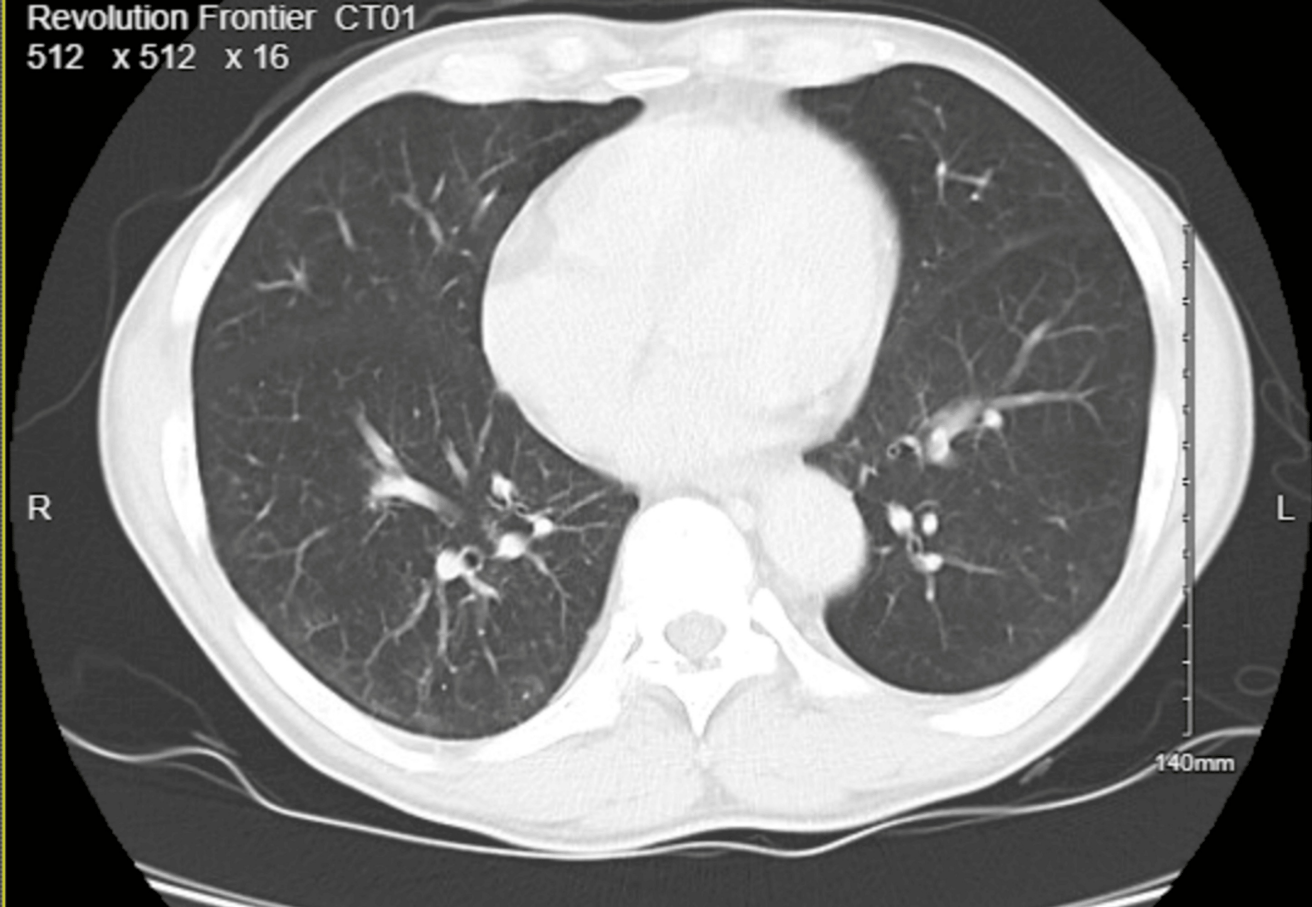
CT chest showing evidence of ground-glass abnormalities, more appreciable in the right lung
